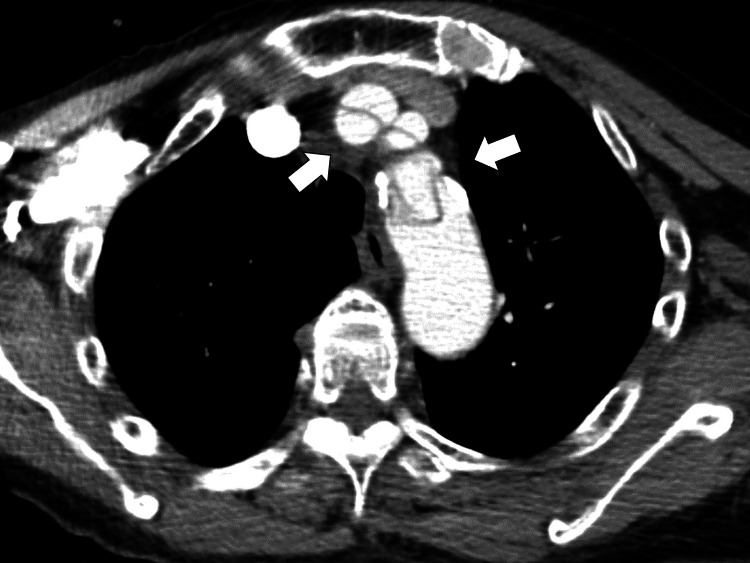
Computed tomography angiography of the chest revealing an ascending aortic dissection extending to the bilateral common carotid arteries (white arrows).
